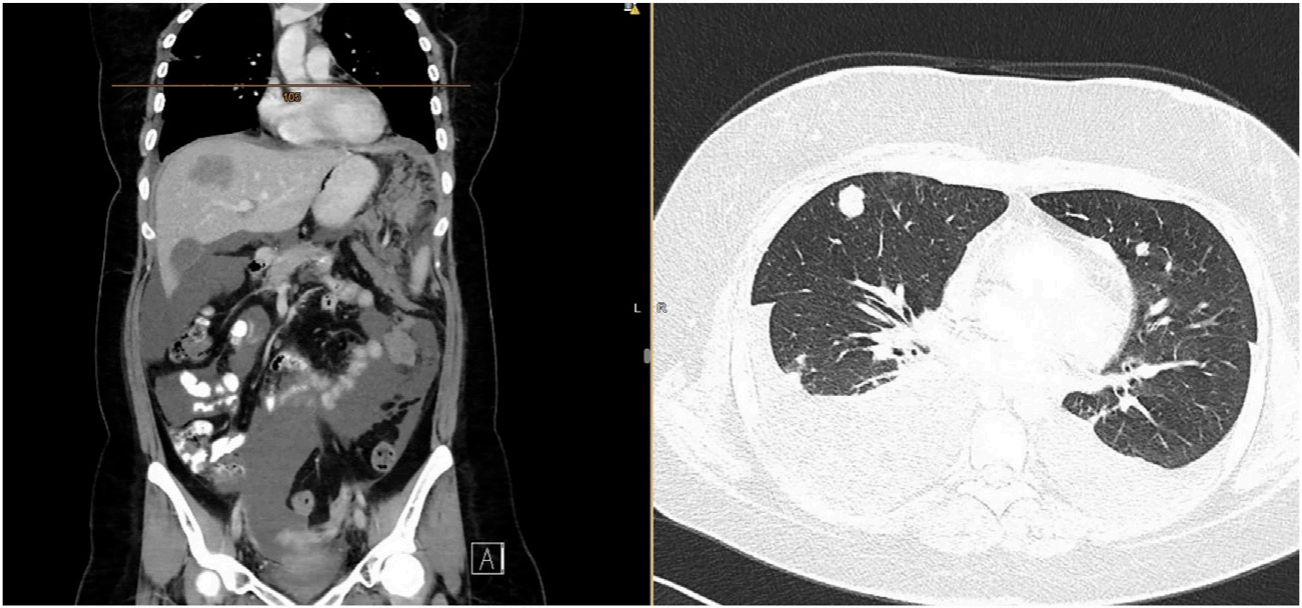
CT scan confirming rapid tumor dissemination with omental, liver, lung, and pleural metastases, with ascites and bilateral pleural effusion.
